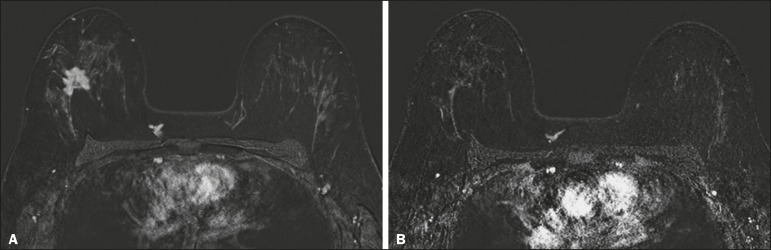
Example of a false-positive result. Pre- and post-NAC MRI (A
